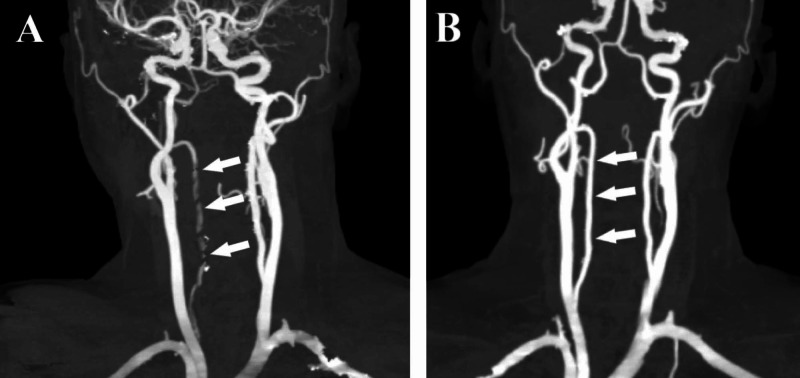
3D maximum-intensity CTA on admission and follow-up CTA three months after discharge – case 1 (A) Three-dimensional maximum-intensity CTA of left vertebral artery on admission showing tapered stenosis (string sign) within V1–V3 segments (arrows). (B) Follow-up CTA after three months showing complete resolution of tapered flow (arrows) CTA: computed tomography angiography
